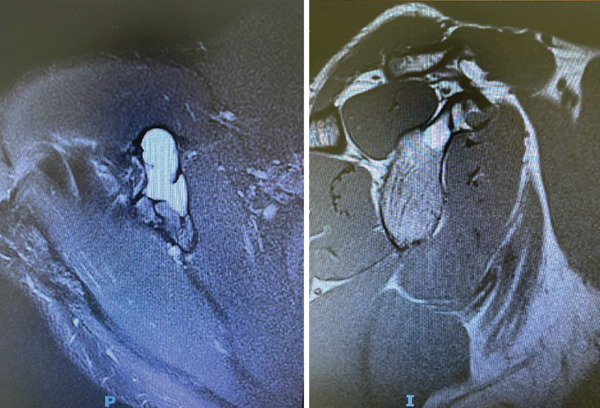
Preoperative magnetic resonance imaging (MRI) of the right shoulder showing a cystic lesion in the coracoid process. The lesion demonstrates heterogeneous signal intensity with multiple internal septations and fluid‐fluid levels, characteristic of an aneurysmal bone cyst. No significant soft tissue extension or surrounding edema is observed.
